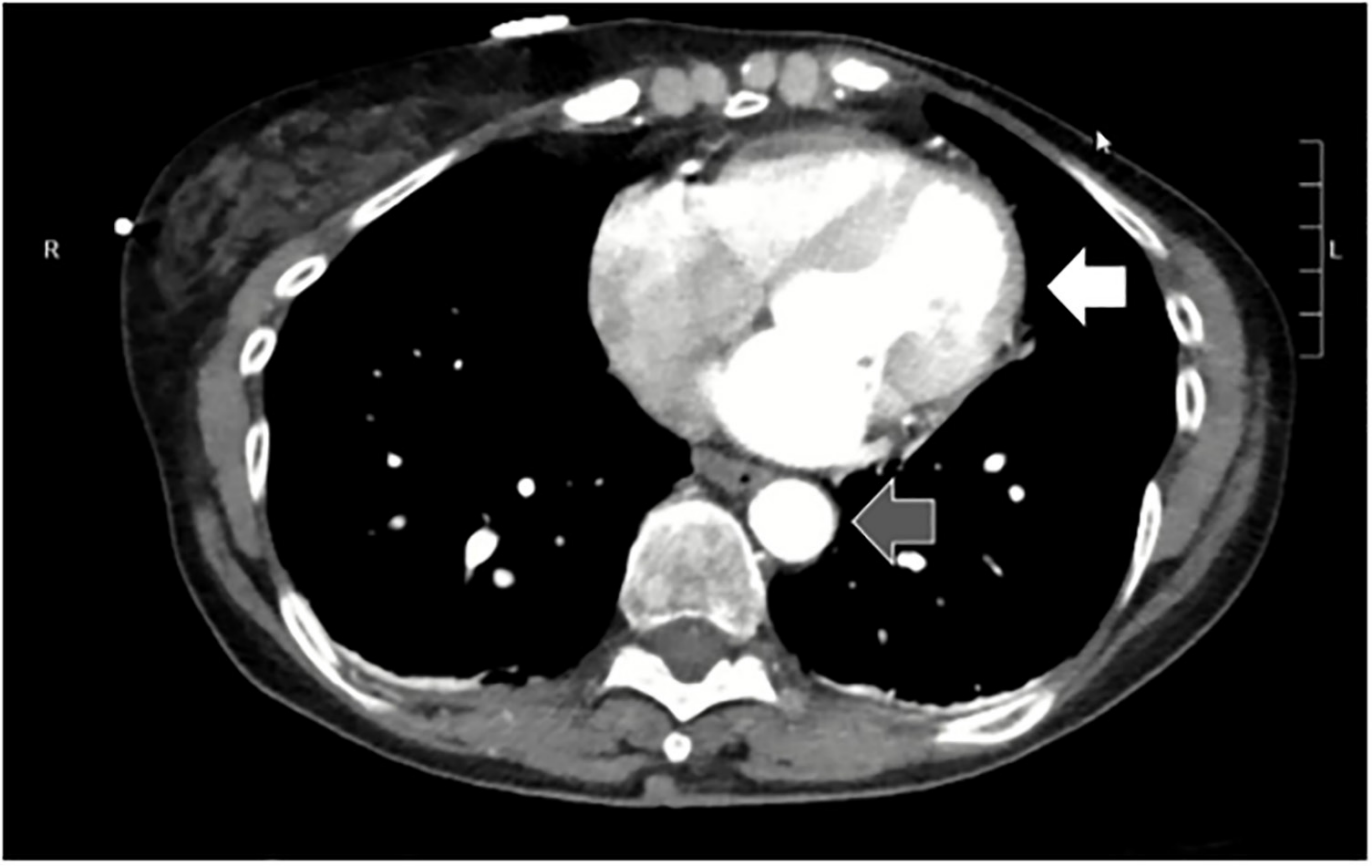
Transverse view of heart (white arrow) and descending aorta (gray arrow) taken from the computed tomography angiogram of the chest of a 73-year-old female with syncope and subsequent motor vehicle collision.
